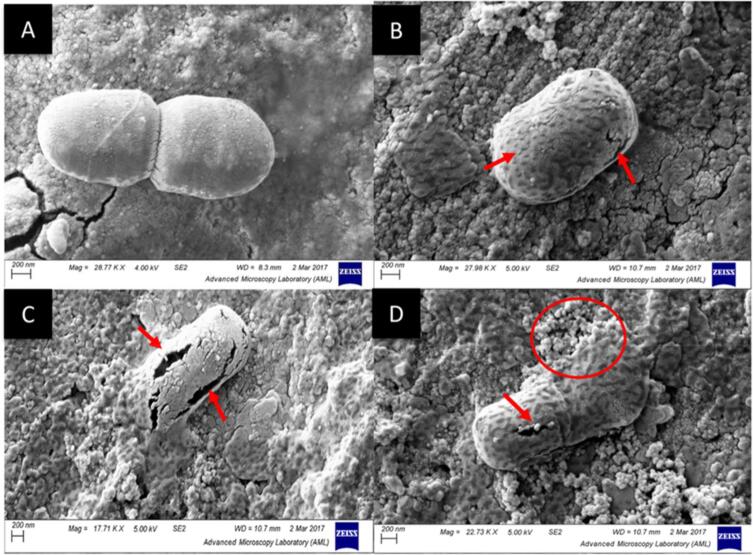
SEM images showing sonoporation due to ultrasonication (a) untreated sample, (b-d) treated sample showing cell rupture and voids after exposure to ultrasound treatment of frequency 20 kHz (Reproduced from Ojha et al., (2018)[26].
